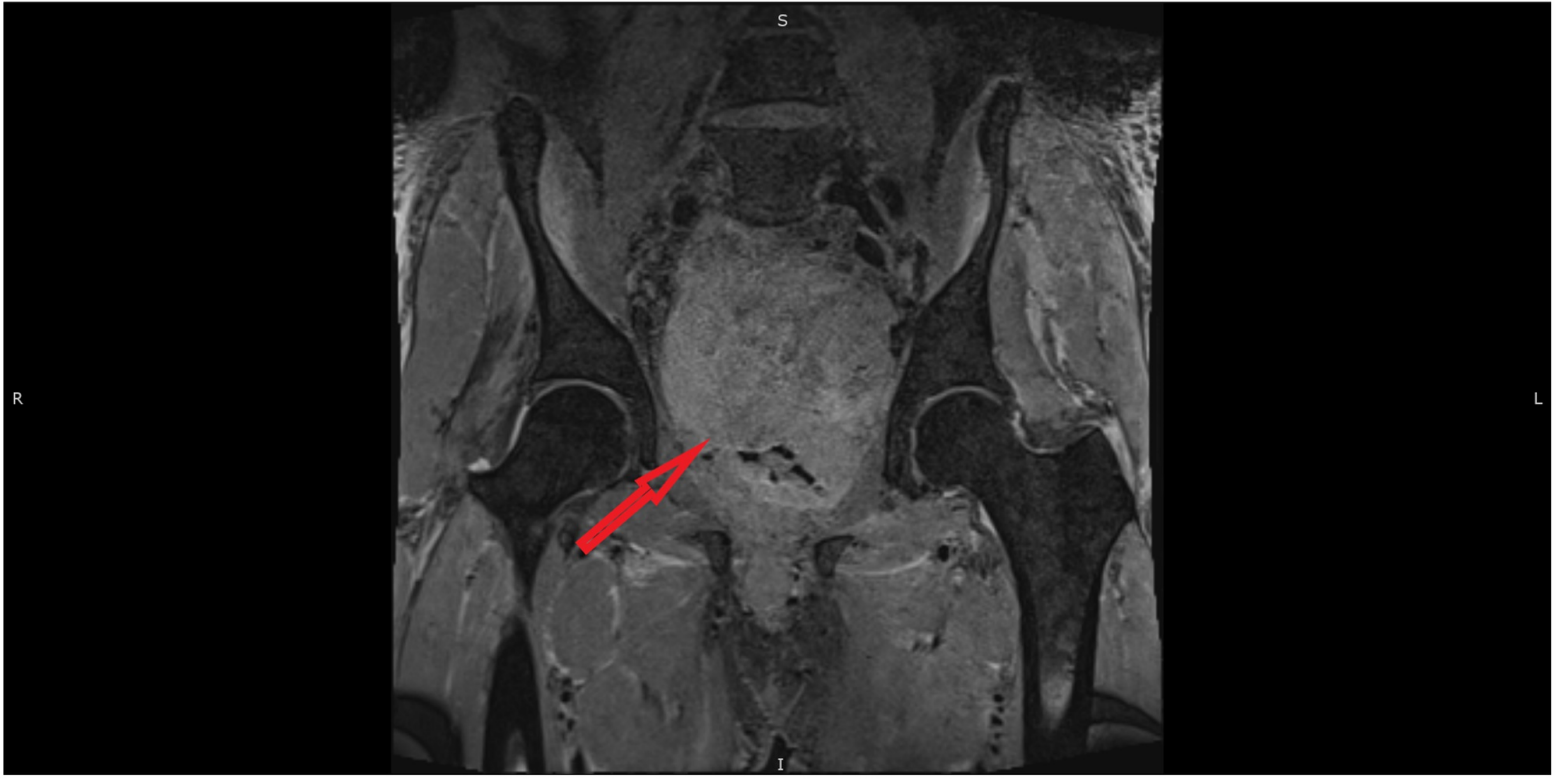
MRI (coronal view) of the pelvis showing large pelvic mass (red arrow)
